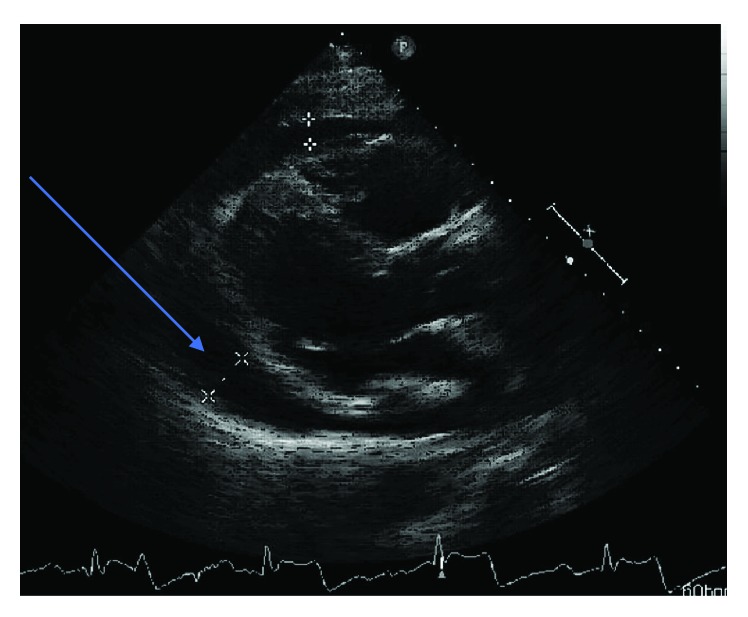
Parasternal long axis view of transthoracic echocardiogram showing fluid within the pericardial space.
